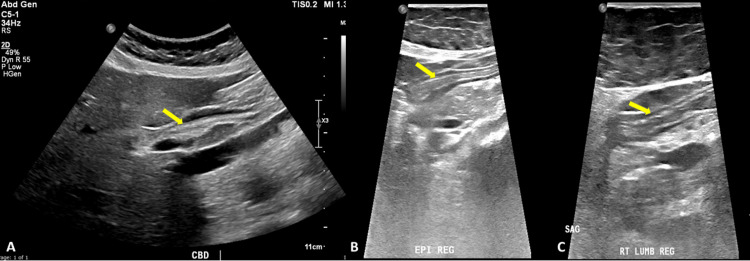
Ultrasound of abdomen showing a linear echogenic structure in the A) CBD, B) epigastric region and C) right lumbar area (yellow arrows).
